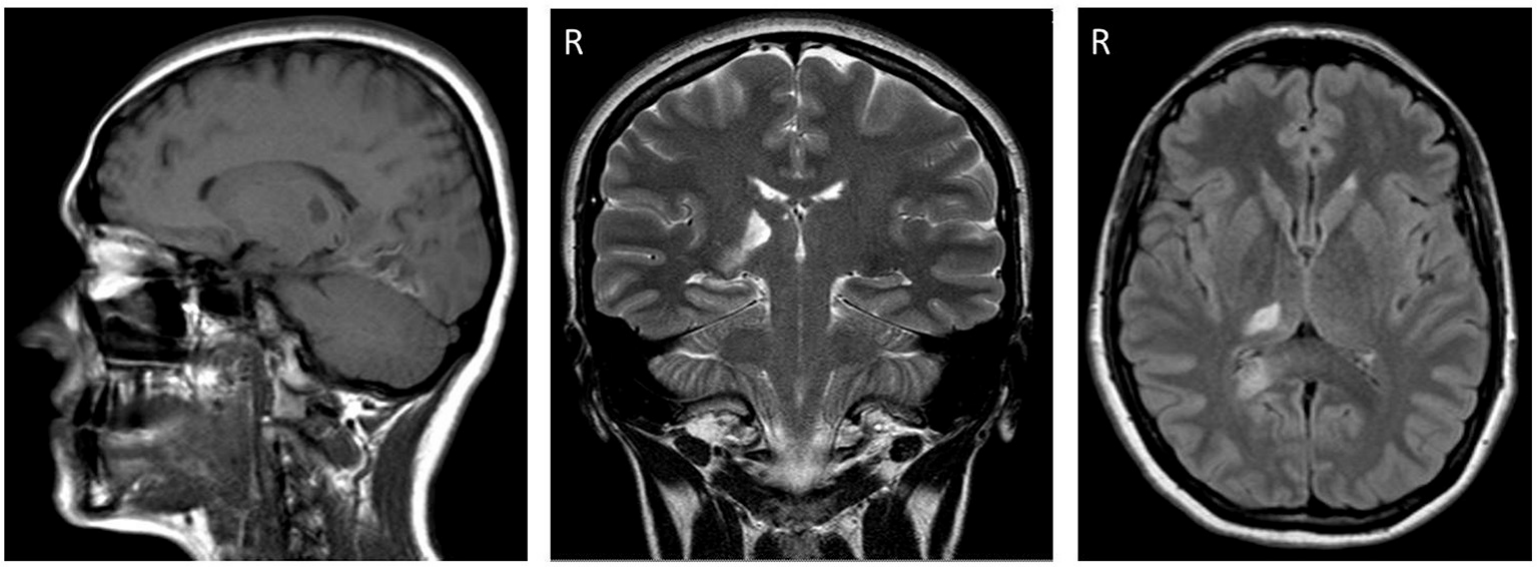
From left to right, sagittal (FLAIR), coronal (T1-weighted), and axial (T1-weighted) sections of brain magnetic resonance imaging (MRI) performed three weeks post-stroke. Note the ischemic lesions affecting the posteroinferior part of the right (R) thalamus and the right occipital lobe.
